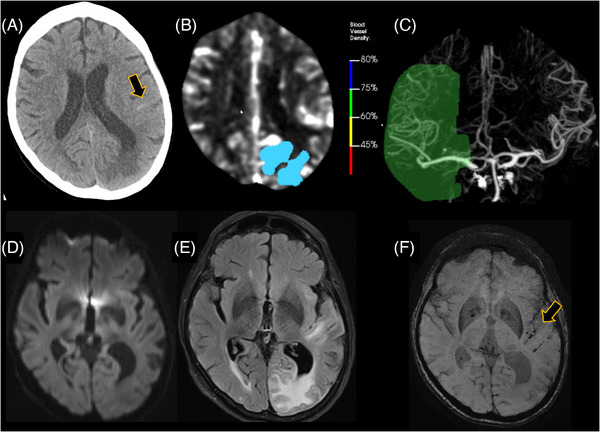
Example of amyloid‐related imaging abnormalities presenting with stroke‐like symptoms in a patient undergoing anti‐amyloid immunotherapy. A woman in her early 70s on anti‐amyloid immunotherapy presented to the ED after awakening with new aphasia, right upper extremity weakness, and confusion. (A) Non‐contrast head CT demonstrated left frontal subarachnoid hemorrhage (arrow) and left parietal vasogenic edema with cortical sparing. (B) In the left parietal lobe, hypodensity between 5 and 12 Hounsfield units (HU) was flagged (blue) by automated stroke assessment software. (C) On CTA, a 60% to 75% decrease in blood vessel density in the left middle cerebral artery distribution was flagged by software (green). (D) Subsequent MRI was negative on diffusion trace (no infarct). (E) MRI FLAIR demonstrated bilateral occipital and left temporal and parieto‐occipital vasogenic edema and sulcal effusions (ARIA‐E). (F) SWI demonstrated superficial siderosis in the left Sylvian fissure and overlying the left temporal lobe (ARIA‐H, arrow). Automated software analysis flagged abnormalities on the CT (B, C); however, these were not infarcts. MRI with diffusion (D) rules out infarct and confirms ARIA (E, F). When patients with MCI or early Alzheimer's disease present in the ED with stroke‐like symptoms, caution is needed when interpreting CT and CTA, even with automated software, which can be misleading, as in this case. ARIA‐E, ARIA manifesting as vasogenic edema; ARIA‐H, ARIA manifesting as hemorrhagic lesions; CT, computed tomography; CTA, CT angiography; ED, emergency department; FLAIR, fluid‐attenuated inversion recovery; MRI, magnetic resonance imaging; SWI, susceptibility weighted imaging; MCI, mild cognitive impairment.
